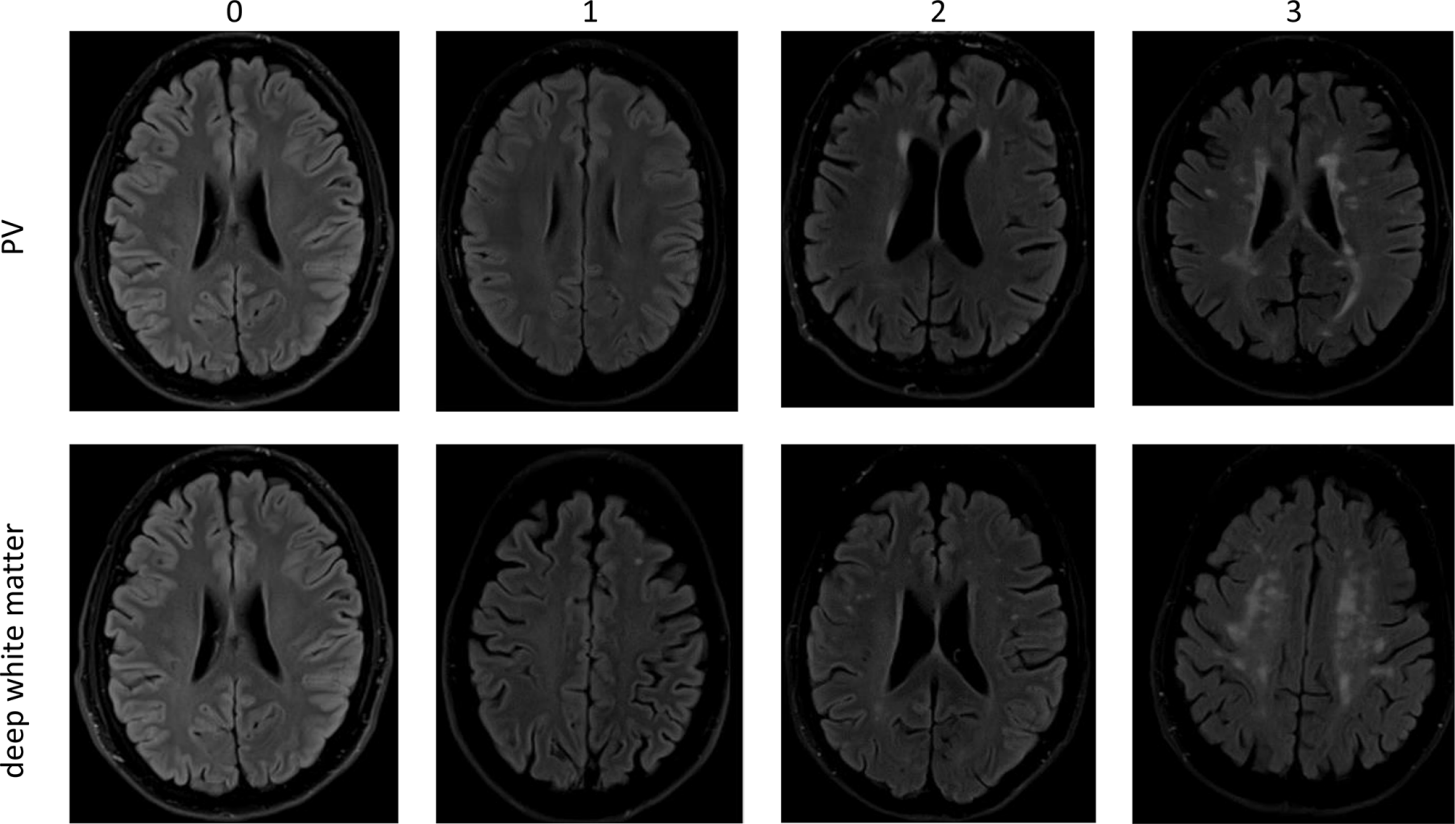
Transverse slice MRI images in FLAIR sequence (fluid attenuated inversion recovery) with examples of periventricular (PV) and deep white matter lesions (modified image from [27]) according to Fazekas et al. [25]
